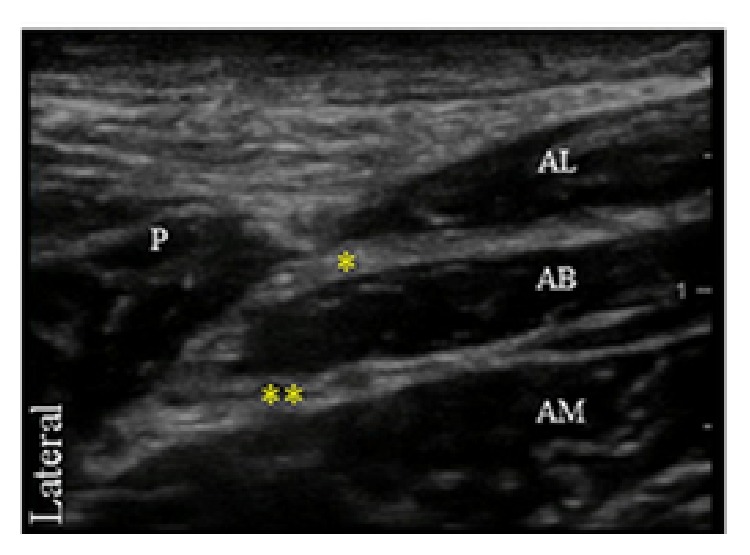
Transverse ultrasound image of the obturator nerve. The distal portion of the obturator nerve divides into an anterior division (single asterisk), identified between the adductor longus (AL) and brevis muscles (AB) and a posterior division (double asterisks) located between adductor brevis and magnus (AM) muscles. Pectineus muscle (P).
